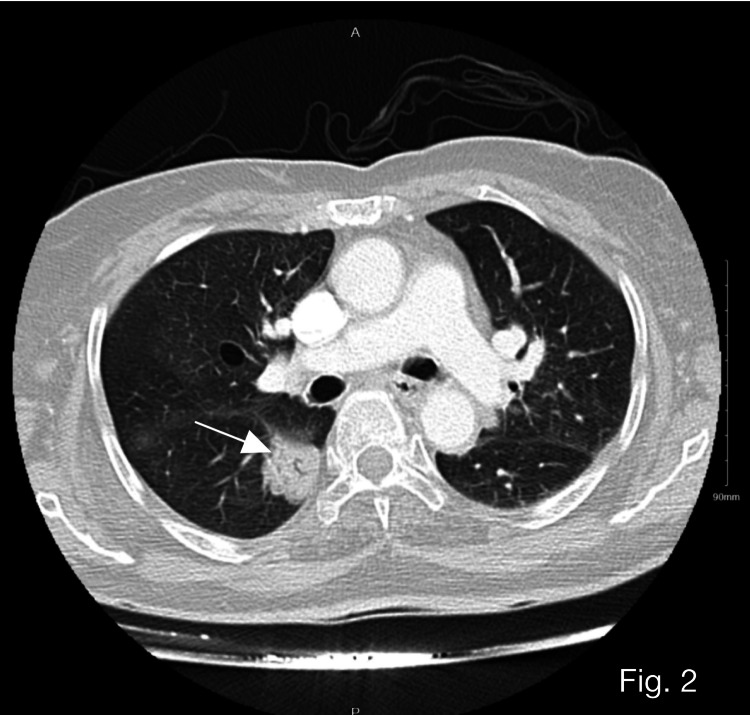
CT of the chest, abdomen, and pelvis (CAP-CT) showing a right lower lobe apical segment mass lesion with air bronchogram extension to the hilum
